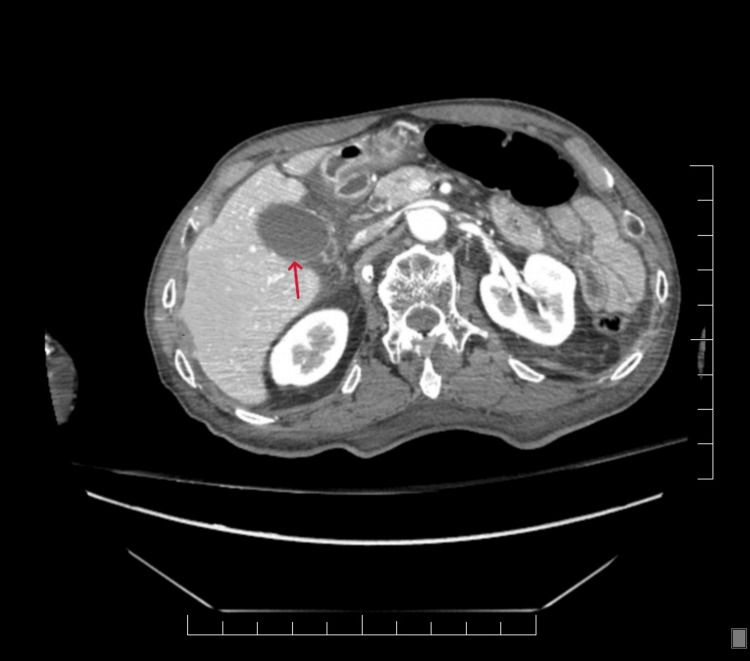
Abdominal computed tomography (CT) imaging Axial contrast-enhanced CT demonstrating a distended gallbladder (red arrow) without gallstones, wall thickening, or pericholecystic fluid.
